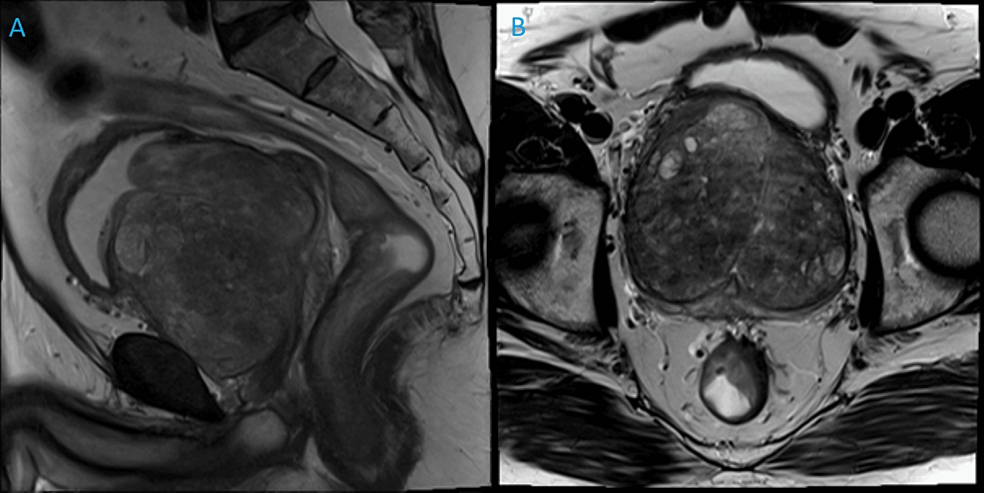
Preoperative prostate MRI showing gland of 372 g with findings of prostatic hyperplasia within the transition zone and no suspicious lesions of prostate cancer. (A) Sagittal prostate MRI. (B) Coronal prostate MRI.
